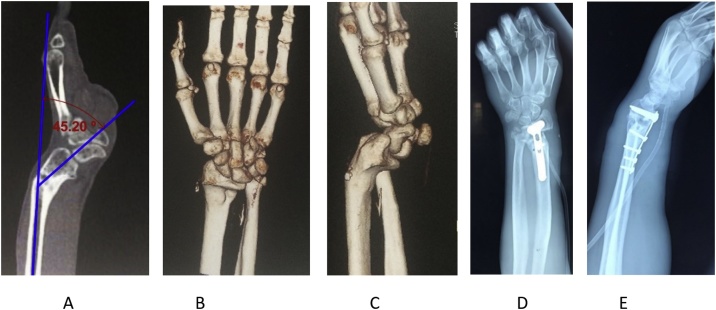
A: The patient’s preoperative CT scans showing malalignment of the distal radius in both sagittal and coronal planes. A: The angle of volar inclination is 45 ° in sagital plane. B: CT scan with reconstruction in the coronal plane and C: in sagital plane. D, E: The first postoperative plain radiographs in a-p and lateral view showing deformity correction.
